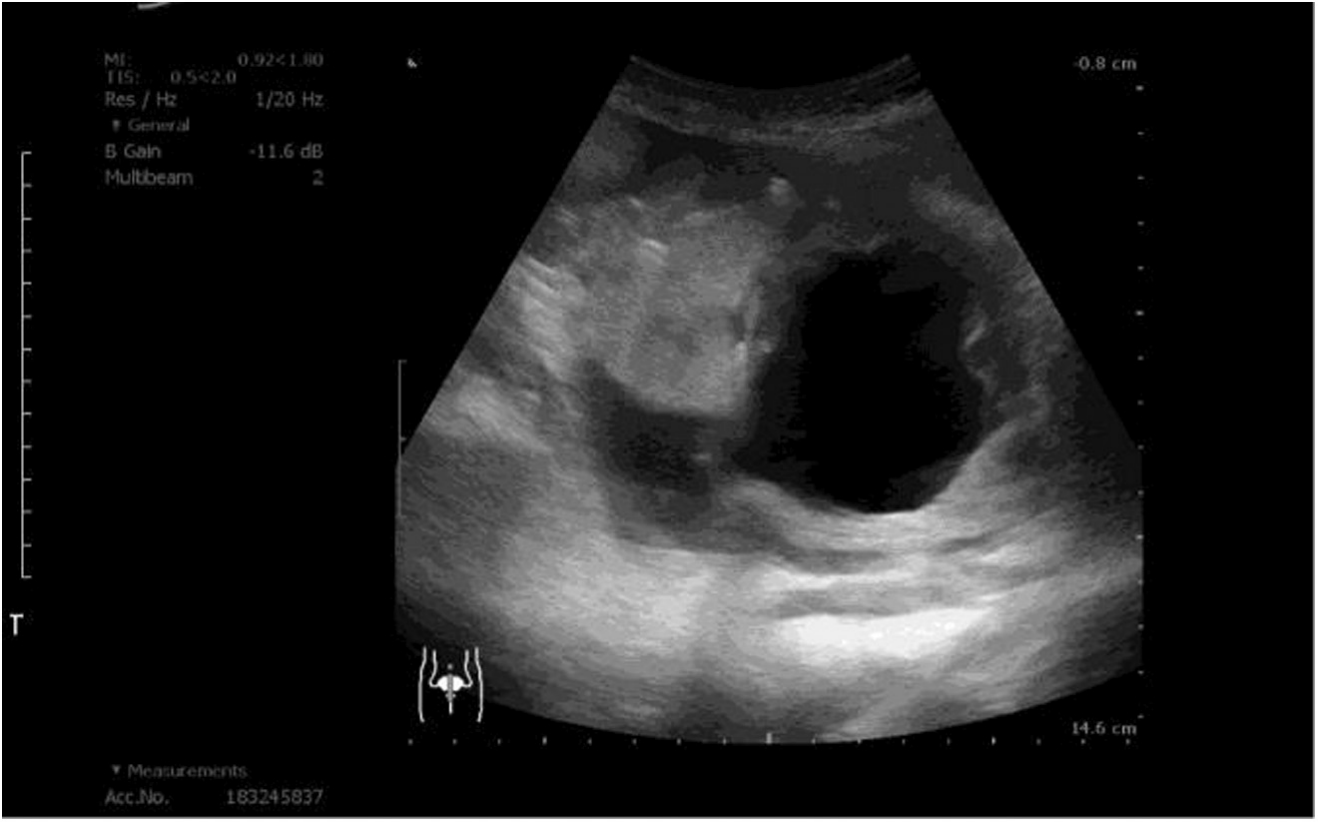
Ultrasound image showing diffuse thickening of the urinary bladder wall that is most notable on the right posterior wall.
